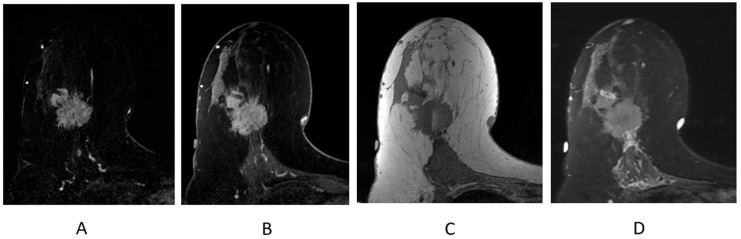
Invasive ductal carcinoma presenting as an irregular mass on breast MRI. (A) Axial post-contrast subtraction, first time point; (B) axial post-contrast T1-weighted fat-suppressed sequence; (C) axial T1-weighted non-fat-suppressed sequence; (D) axial STIR sequence.
